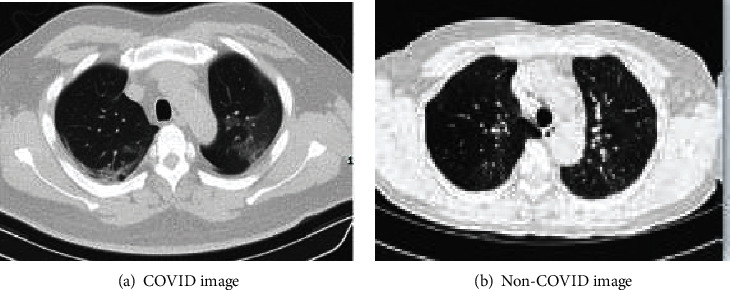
Example of CT images for COVID and non-COVID.
